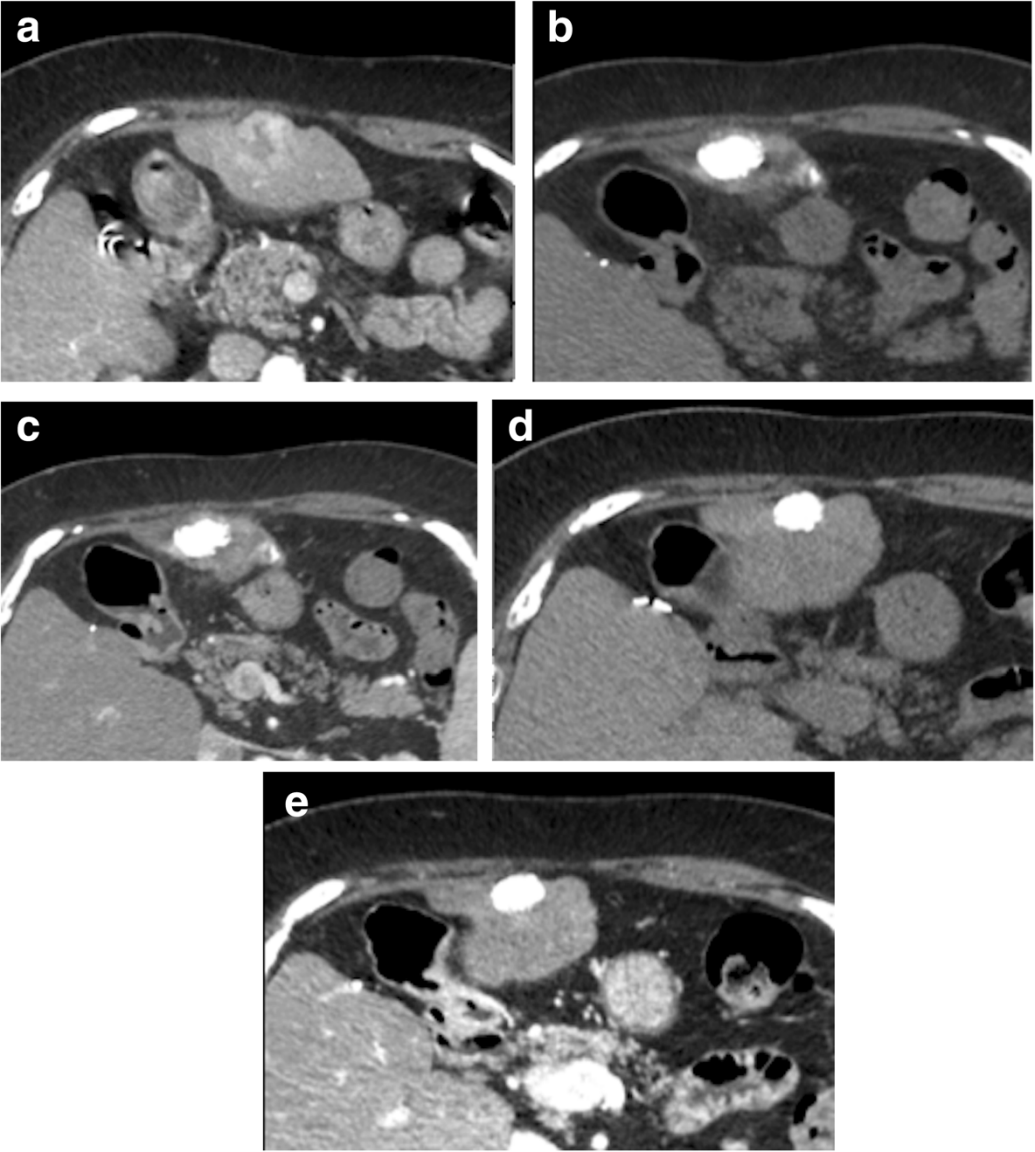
Absence of local progression of an HCC showing a complete response according to mRECIST with complete lipiodol retention in an 83 yr-old male with HBV related cirrhosis. a baseline CT image obtained during hepatic arterial phase (HAP) shows a hyperenhancing HCC in the left liver lobe. b and c first follow-up CT images showing the lesion presenting with complete lipiodol retention on the precontrast image (b) and no residual enhancement on HAP (c). d-e 24 months follow-up CT images obtained during unenhanced (d) and HAP (e) show that the nodule has decreased in size with persistent complete lipiodol retention in the lesion. No enhancing lesion in noticed on HAP (e)
